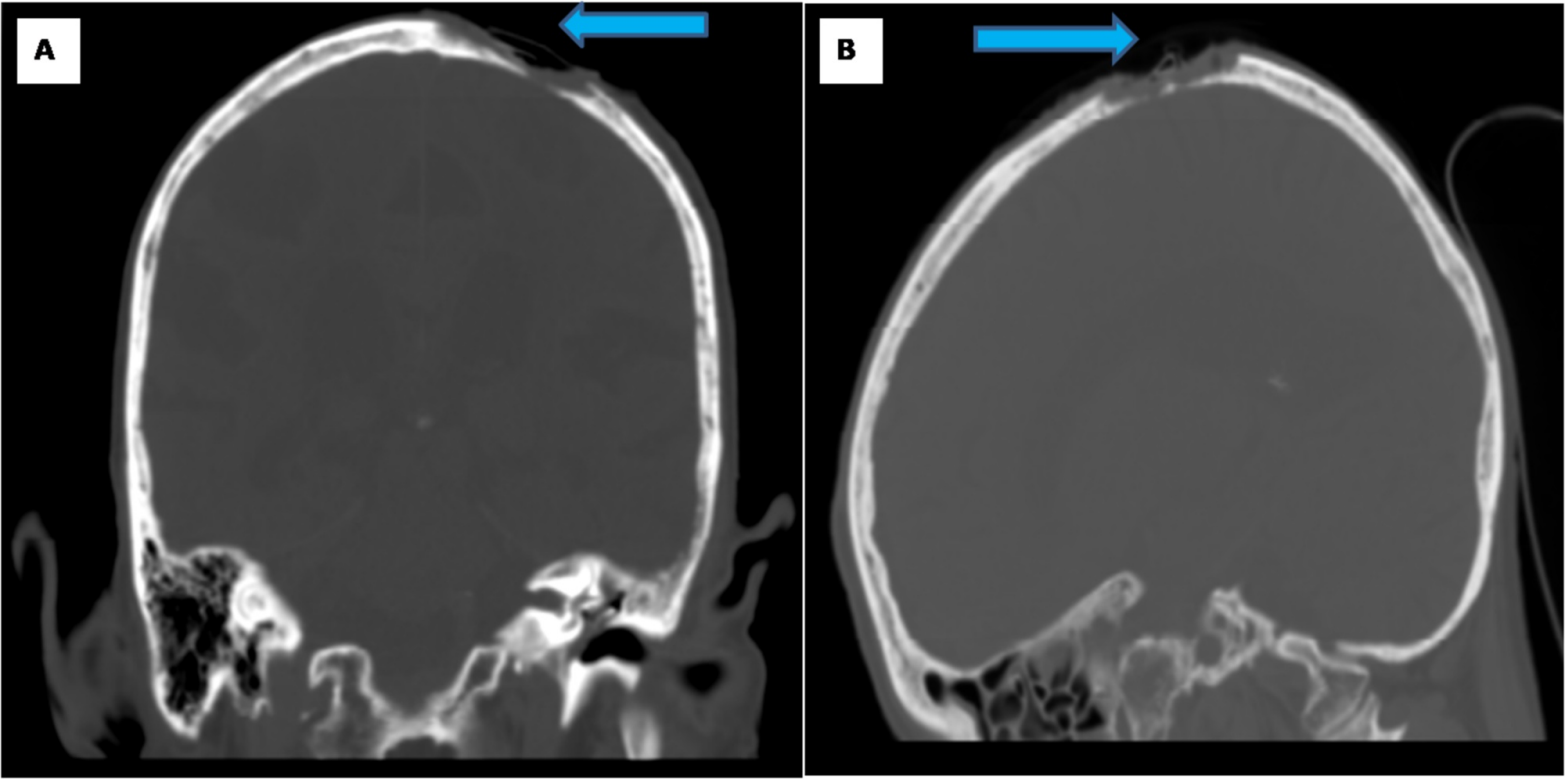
CT scan of the head There is a destructive lytic lesion involving the adjacent calvarium with severe thinning/destruction of the inner table (blue arrow). (A) Coronal view, (B) sagittal view.
